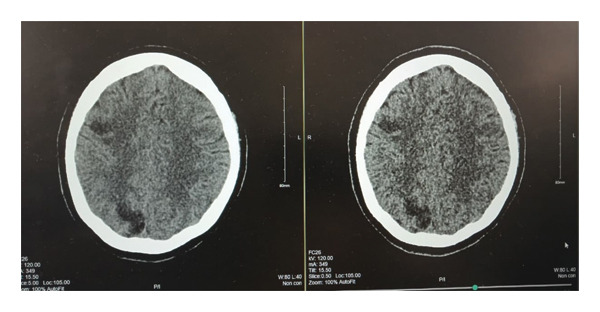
Computed tomography (CT) brain of the current patient showing a hypodense lesion approximately 2 ∗ 3 cm in right occipital lobe that is suspected suboccipital infarct. In right fronto‐occipital infarct, there is 2 ∗ 2 cm hypodense lesion suspecting an acute ischaemic infarct.
